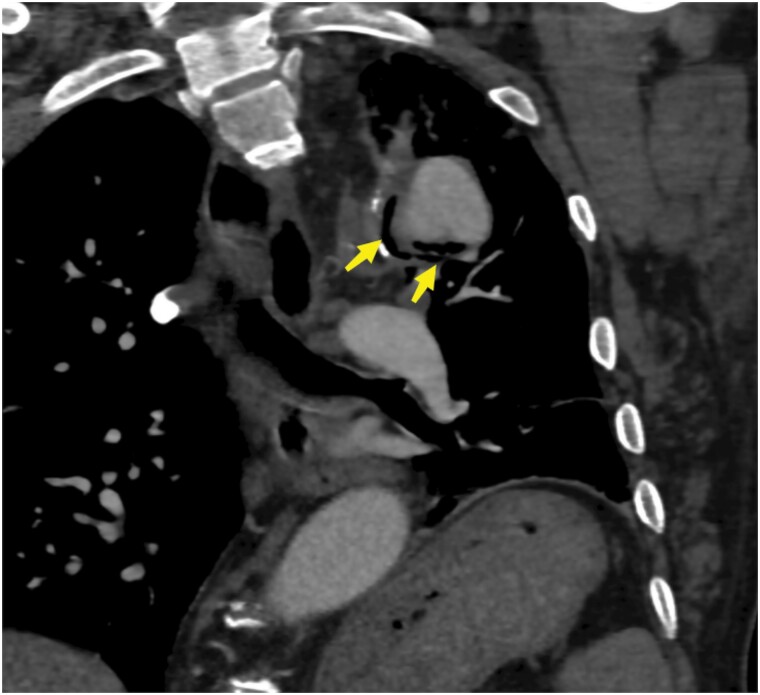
CT angiography in the mediastinal window shows a prosthetic fistula involving the lung.
